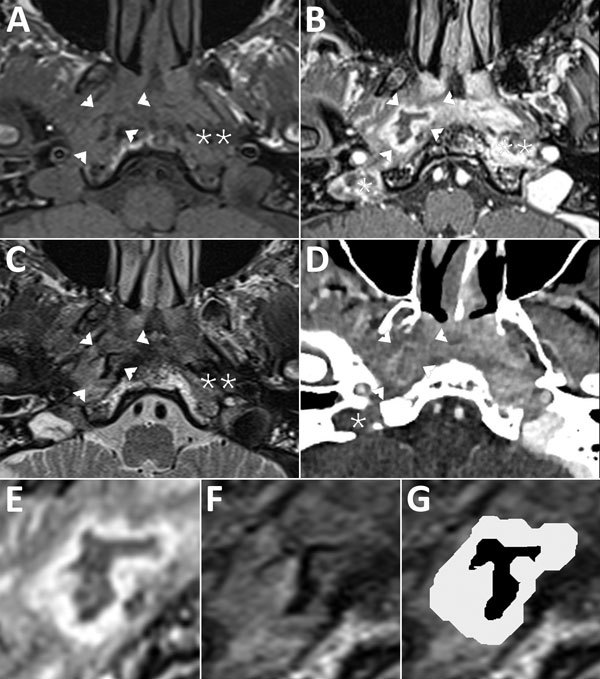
Magnetic resonance imaging (MRI) of a patient with malignant otitis externa, France. Cross-sectional imaging demonstrates a central skull base osteomyelitis in patient’s temporal bone. A) T1-weighted imaging; B, E) 3-dimensional T1-weighted imaging with gadolinium enhancement and fat saturation; C, F, G) T2-weighted imaging; and CT with iodine enhancement (D). Single asterisks (*) indicate jugular bulb thrombosis (panels B, D); double asterisks () indicate deep-spaces cellulitis (panels A–C). Arrowheads indicate parapharyngeal abscess at right (panels A–D); parapharyngeal abscess is also visible as a gray layer (panels E, G). The content of the abscess has an unusual “ink smudge” pattern with no signal in T2-weighted imaging, visible as a black layer (panels F, G). This pattern is consistent with a mycetoma surrounded by granulation tissue.
